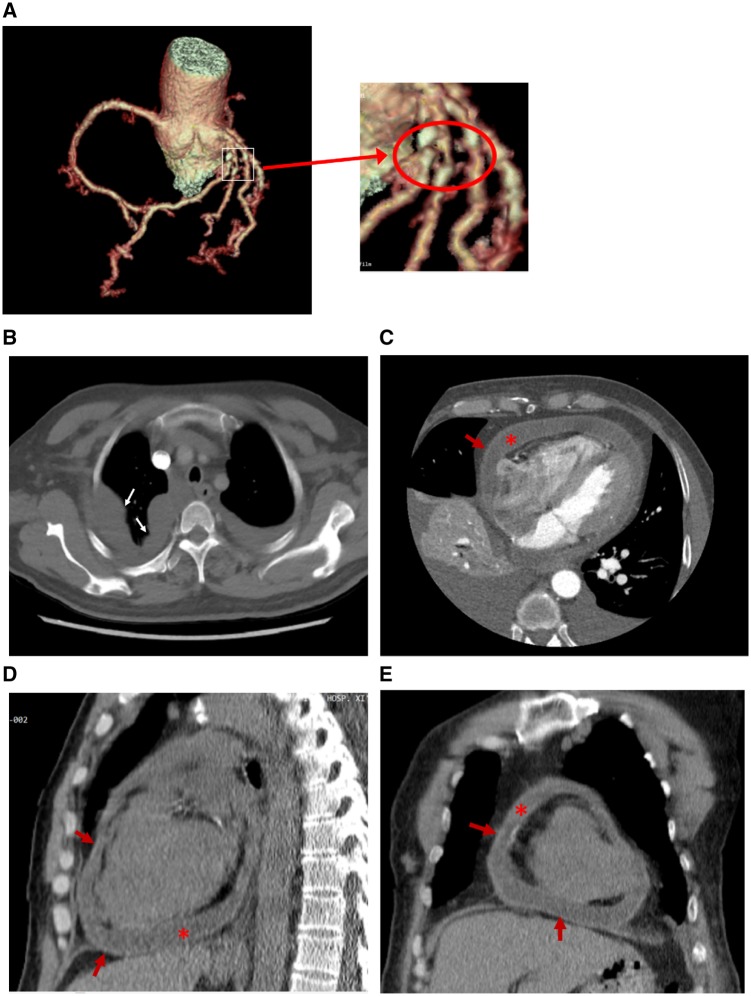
Computed tomography angiography scan on admission showed severe stenosis of the diagonal branch of left anterior descending artery (*A*), pleural effusion (*B*), mild pericardial effusion and the thickening pericardium (*C–E*). However, all evidences of the thickening pericardium were ignored by the radiologist. White arrows indicate irregular fluid levels and encapsulated effusion, supporting the chronic process. Red arrows indicate thickening pericardium. The pericardial thickness by high-resolution computed tomography was more than 4.5 mm. Red asterisks indicate pericardial effusion.
